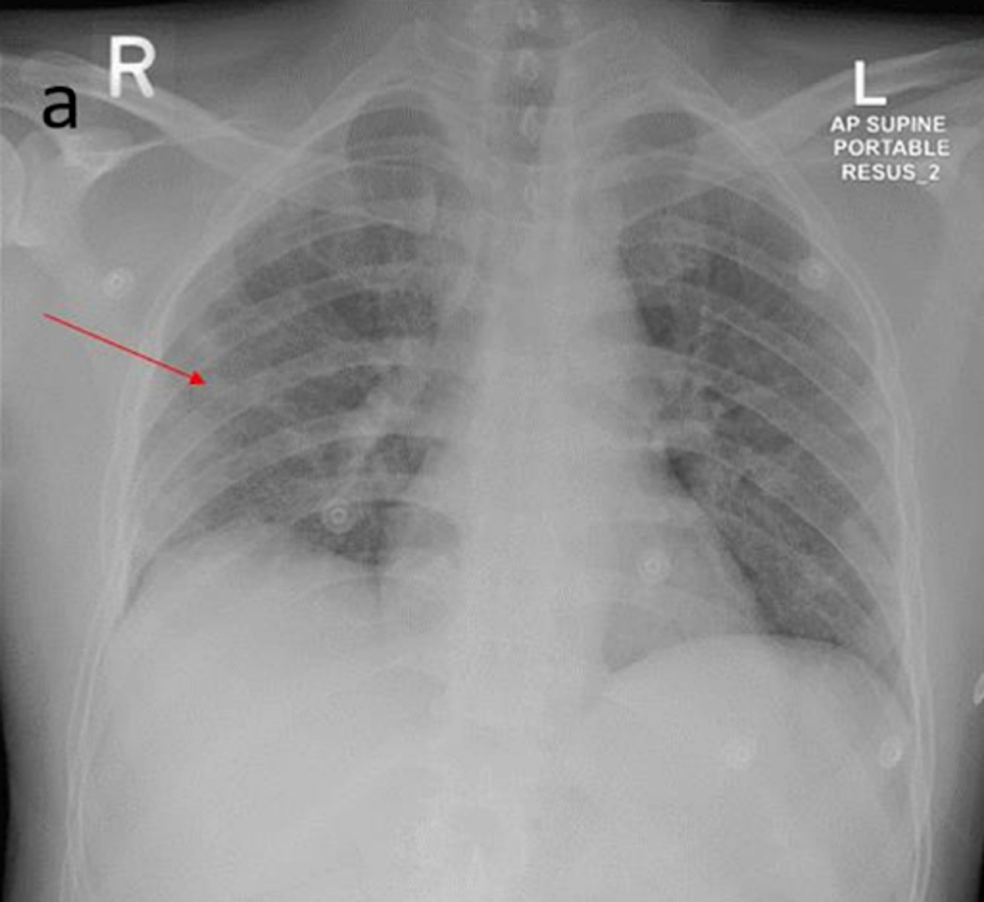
Chest X-ray (AP) of COVID-19 patient Chest X-ray shows patchy peripheral ground-glass opacities in the bilateral lung fields.
